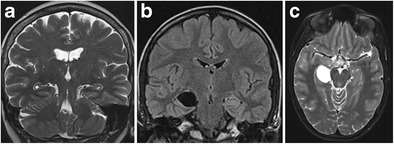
Bilateral sulcal remnant cysts (a) and right-sided choroid fissure cyst (b, c). Coronal T2 shows small bilateral cysts at the apex of the hippocampal fold between the dentate gyrus and Ammon’s horn (a). Coronal FLAIR (b) and axial T2-weighted (c) images show a space-occupying cystic lesion, iso-intense to cerebrospinal fluid, at the level of the right choroid fissure
